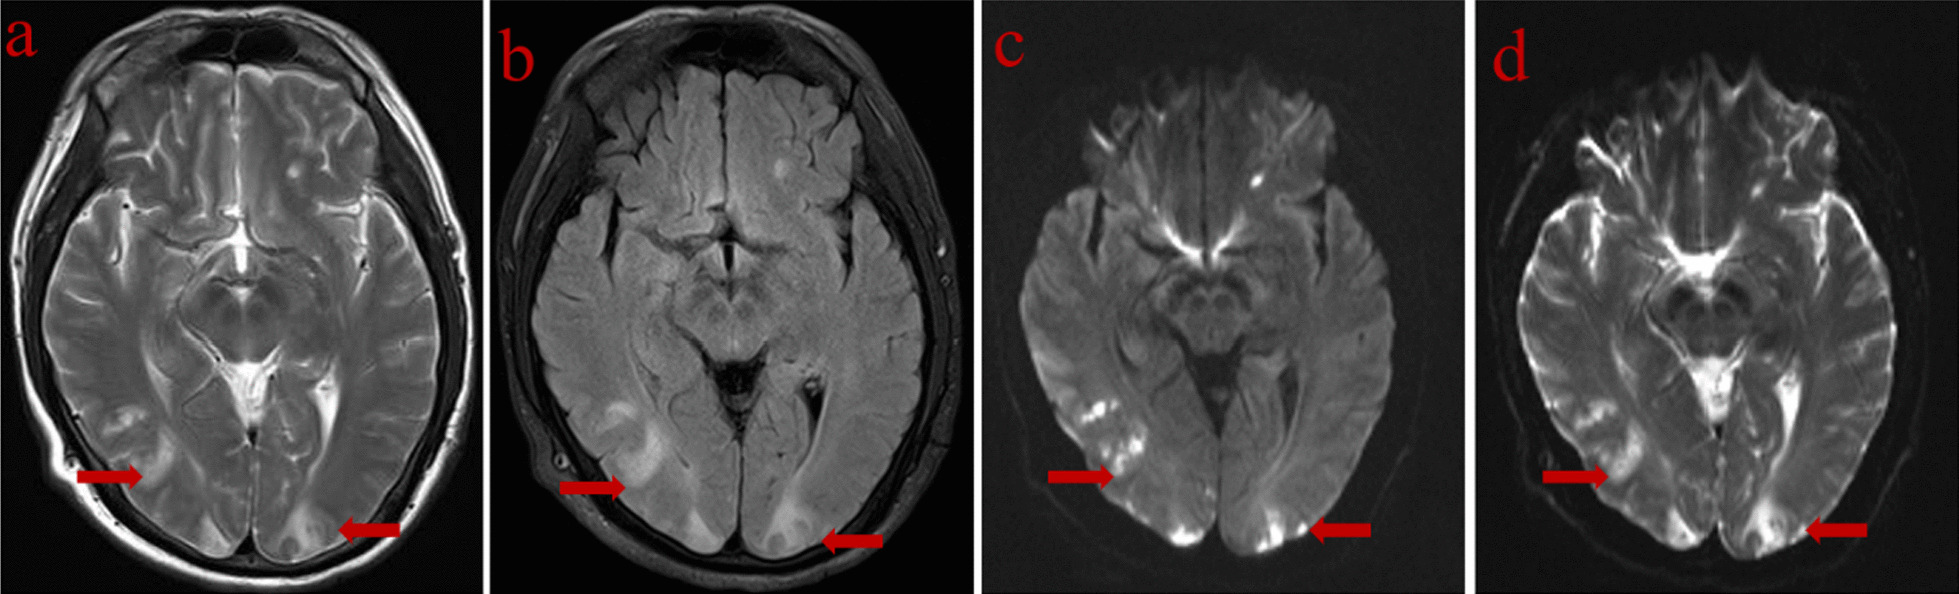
The brain MRI scan showed multiple patchy abnormal signal in the bilateral temporal lobe and the bilateral occipital lobe. T1WI (a),T2-FLAIR (b), DWI (c), ADC (d)
